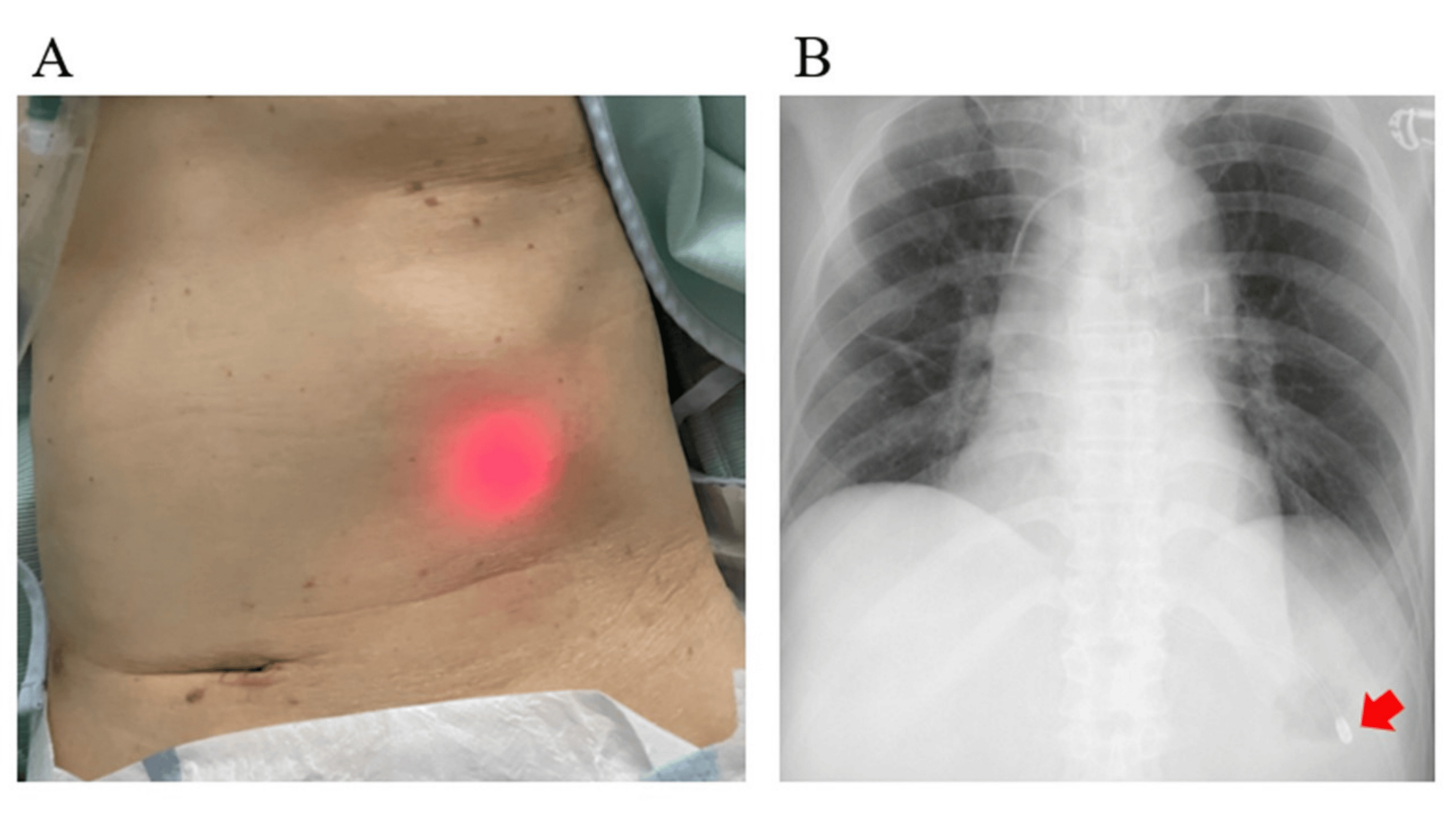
Positive BTI light and X-ray image (case 4) (A) The BTI light was detected in the epigastric area. (B) Corresponding X-ray image. The arrow indicates the NGT tip. BTI, biologically transparent illumination; NGT, nasogastric tube
